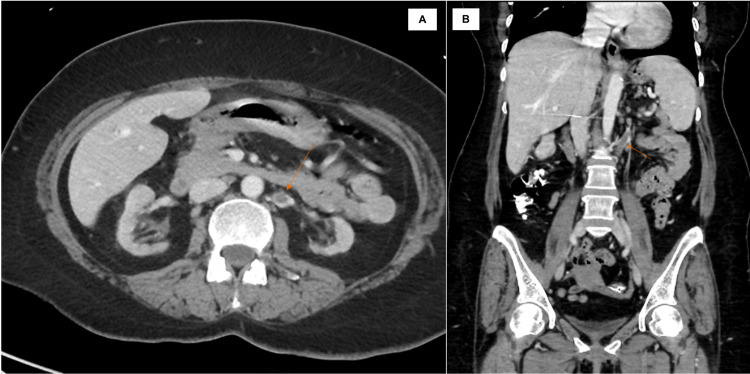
A &B: Axial and sagittal contrast-enhanced CT showing left renal vein Thrombosis (arrow) and resolution of the renal masses.
